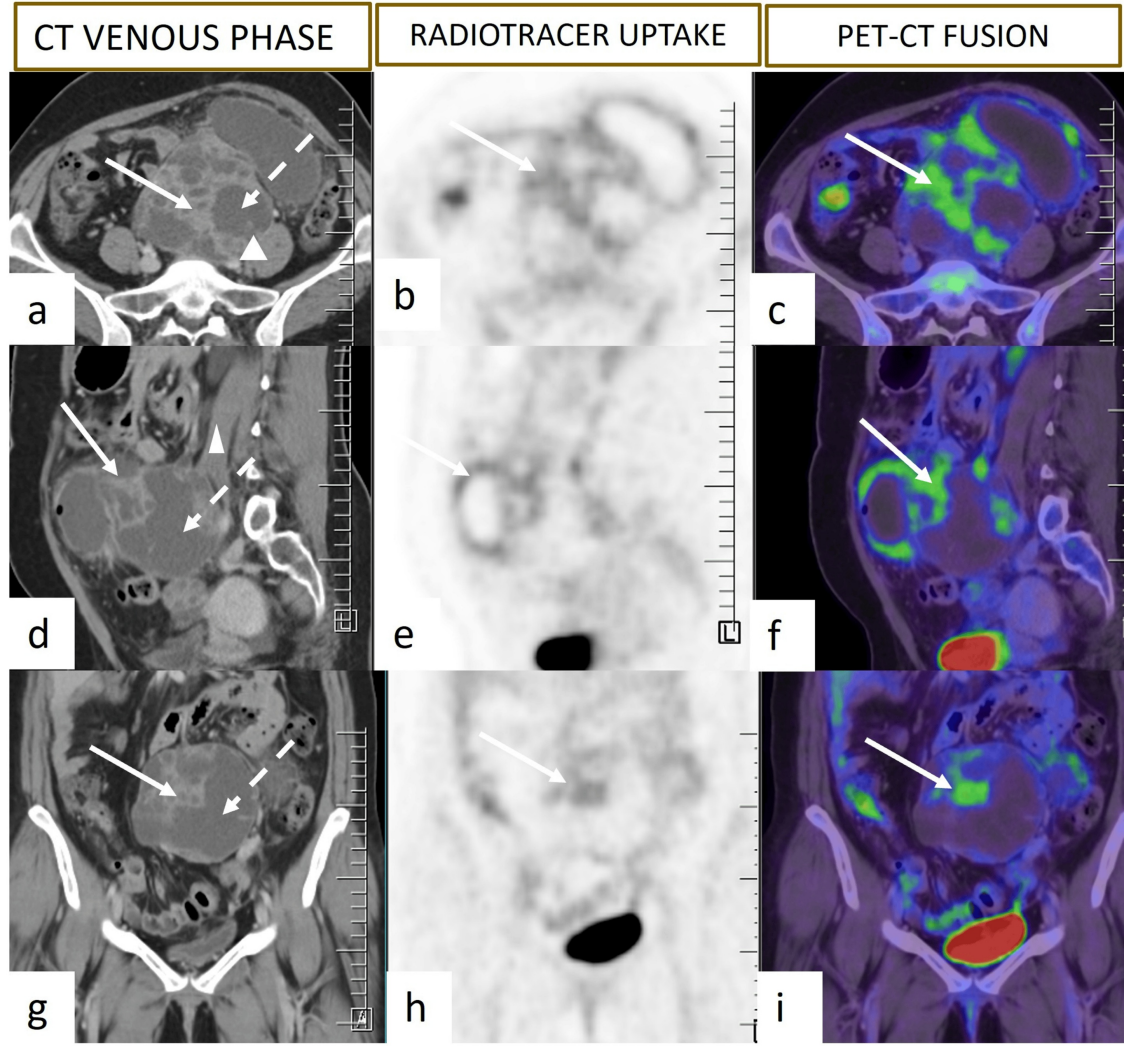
Axial (a, b, and c) and reformatted coronal (d, e, and f) and sagittal (g, h, and i) PET-CT images show a large, FDG-avid (SUVmax: 3.66) multiloculated solid cystic lesion in the mesentery. The solid components are enhancing and FDG-avid (arrow) with central non-enhancing necrotic areas (dashed arrow) within. The lesion is seen abutting the left psoas muscle (arrowhead) and adherent to the sigmoid colon. PET-CT: positron emission tomography-computed tomography, FDG: fluorodeoxyglucose, SUVmax: maximum standardized uptake value
